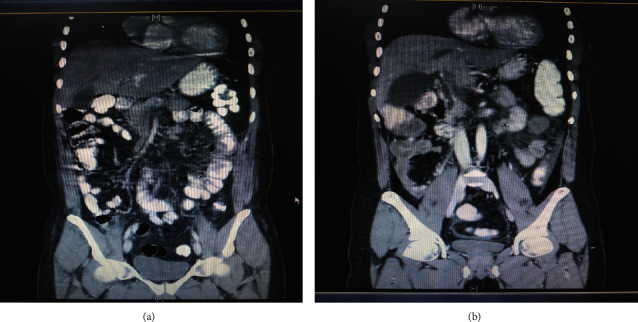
CT abdomen with IV contrast showing mesenteric vein thrombosis and thickening of the small bowel with skipped lesions.
